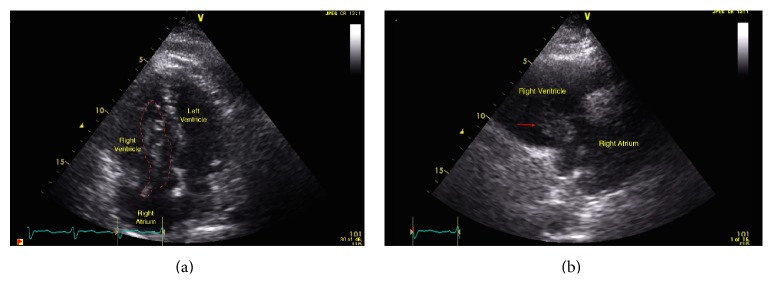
(a) An apical 4-chamber echocardiographic view of the heart showing the right heart thrombus protruding through the tricuspid valve. (b) A right ventricular inflow echocardiographic view of the heart showing the right heart thrombus protruding through the tricuspid valve.
